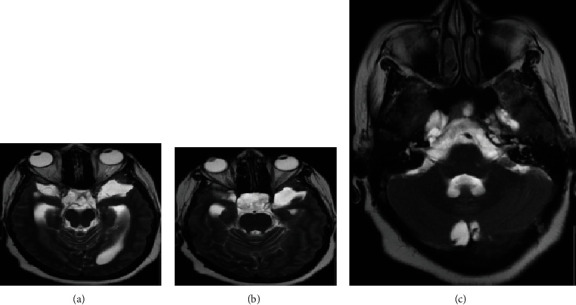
Axial view of a T2-weighted image of the midbrain (a) and upper pons lower midbrain (b) levels showing expanded temporal horns, increase in the subarachnoid space of the optic nerves, optic nerves' tortuosity, and deviation of the optic chiasm towards the left side, all indicating high ICP. The middle pons level shows bilateral Meckel's cave expansion and scalloping of the right petrous apex associated with a cephalocele (c).
